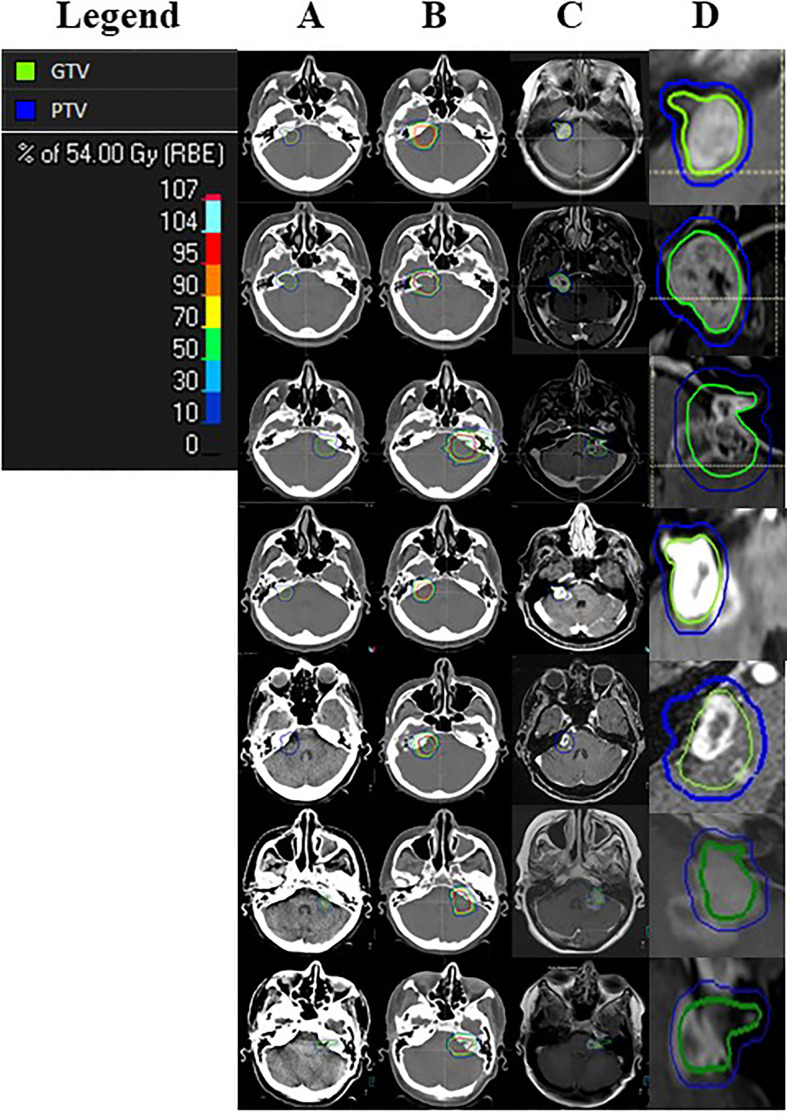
Cases of Radiation-induced Contrast Enhancements (RICE). The image presents all seven cases of RICE observed in our study cohort. Column A presents the planning computer tomography with gross target volume (GTV, green) and planning target volume (PTV, blue) for each patient. Column B demonstrates the treatment plan with isodoses for each patient. Column C presents the follow-up magnetic resonance imaging (MRI) with the observed RICE at the time of its first notice and a projection of the initial GTV and PTV in this MRI with an enlargement of this region (column D).
